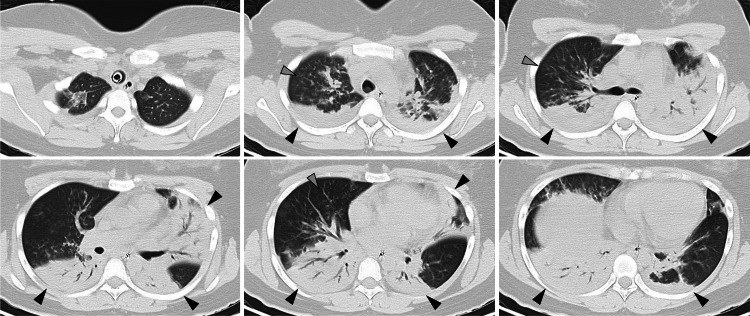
Chest computed tomography on the day of admission showing exacerbated bilateral consolidations (black arrowheads) and centrilobular nodules in the right lung (gray arrowheads)
